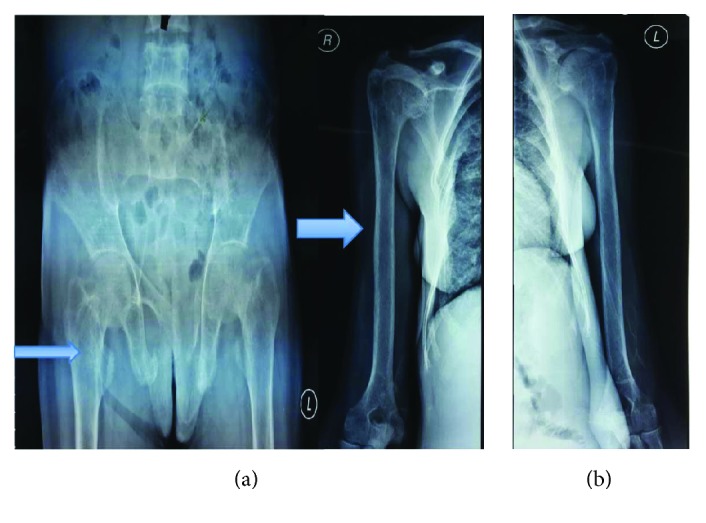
Case 1. Bone survey radiographic examination of the (a) pelvis showing multiple lytic lesion along the bilateral iliac bone and pubic bone and the (b) humerus and clavicle showing multiple lytic lesion along the lateral edge of the humerus. Pathologic fractures of the distal part of the left clavicle are observed, increased bone resorption among the bilateral clavicle.
